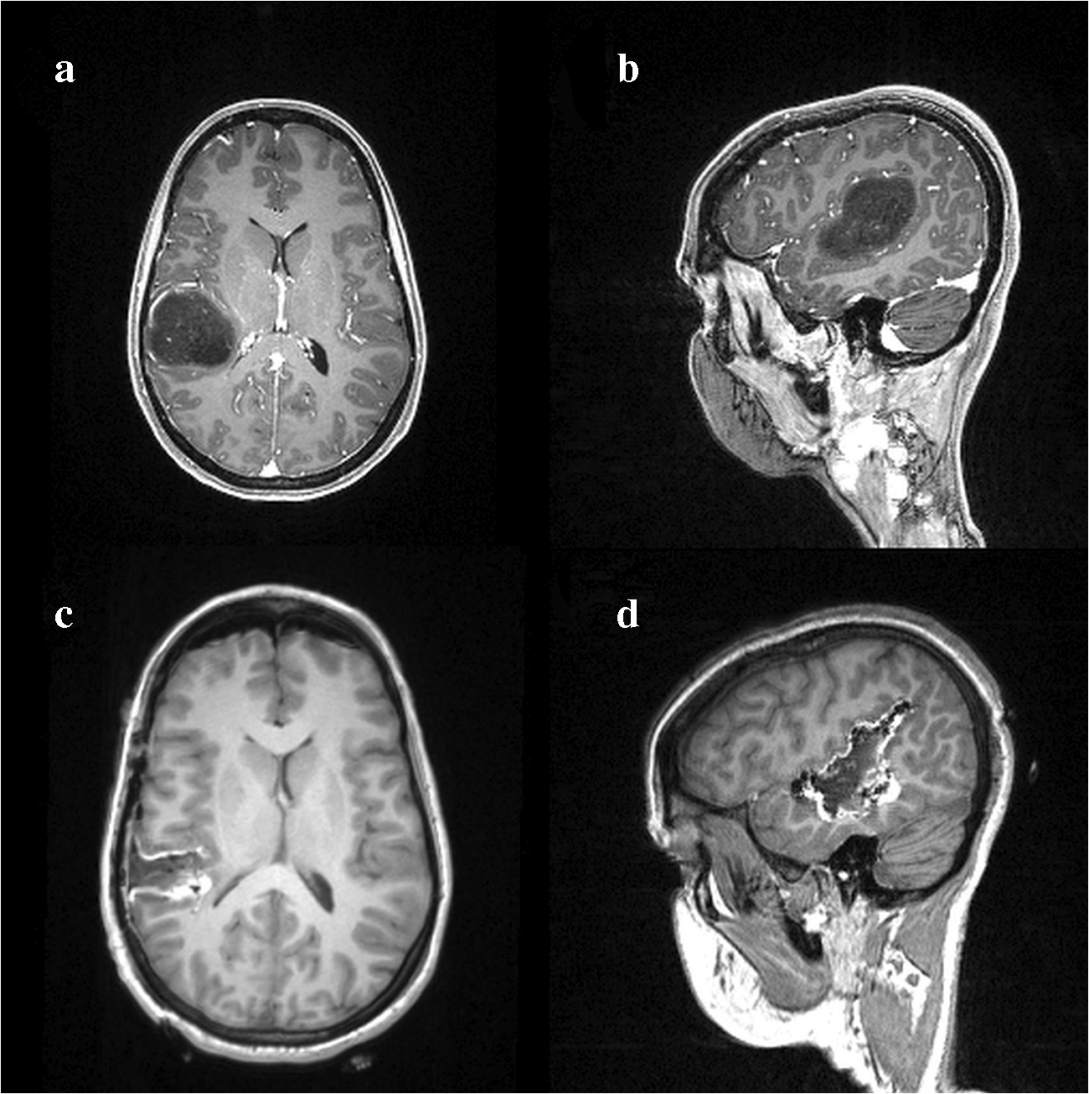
a, b T1-weighted contrast-enhanced MRI scan at preoperative phase demonstrate typical appearance of a low-grade glioma without any accumulation of the contrast medium. c, d T1-weighted contrast-free follow-up MRI scan 24 h after surgery revealed gross total removal of the tumor with thin hyperintense line indicative of minimal blood accumulation in the tumor bed
